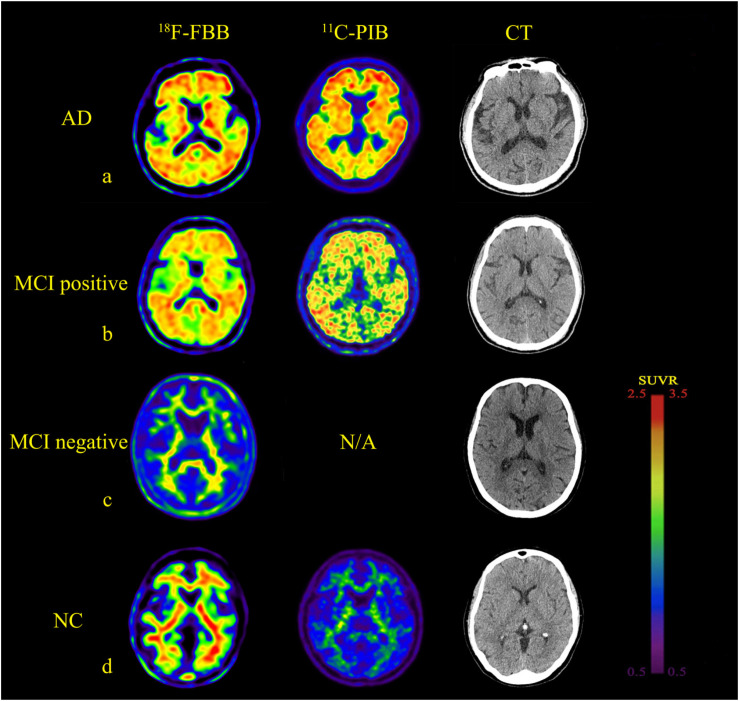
Comparison of brain axial PET images of ^18^F-FBB and ^11^C-PIB in NC, MCI, and AD for example. (a) NC participant (75-years-old, male, MMSE = 28). (b) MCI patient (73-years-old, male, MMSE = 27). (c) MCI patient (67-years-old, female, MMSE = 24). (d) Early stage AD patient (78-years-old, male, MMSE = 24). Both ^18^F-FBB PET/CT and ^11^C-PIB PET/CT images showed no amyloid deposition (a,c). Amyloid deposition in widespread cortical was almost identical in ^18^F-FBB PET/CT and ^11^C-PIB PET/CT (b,d).
